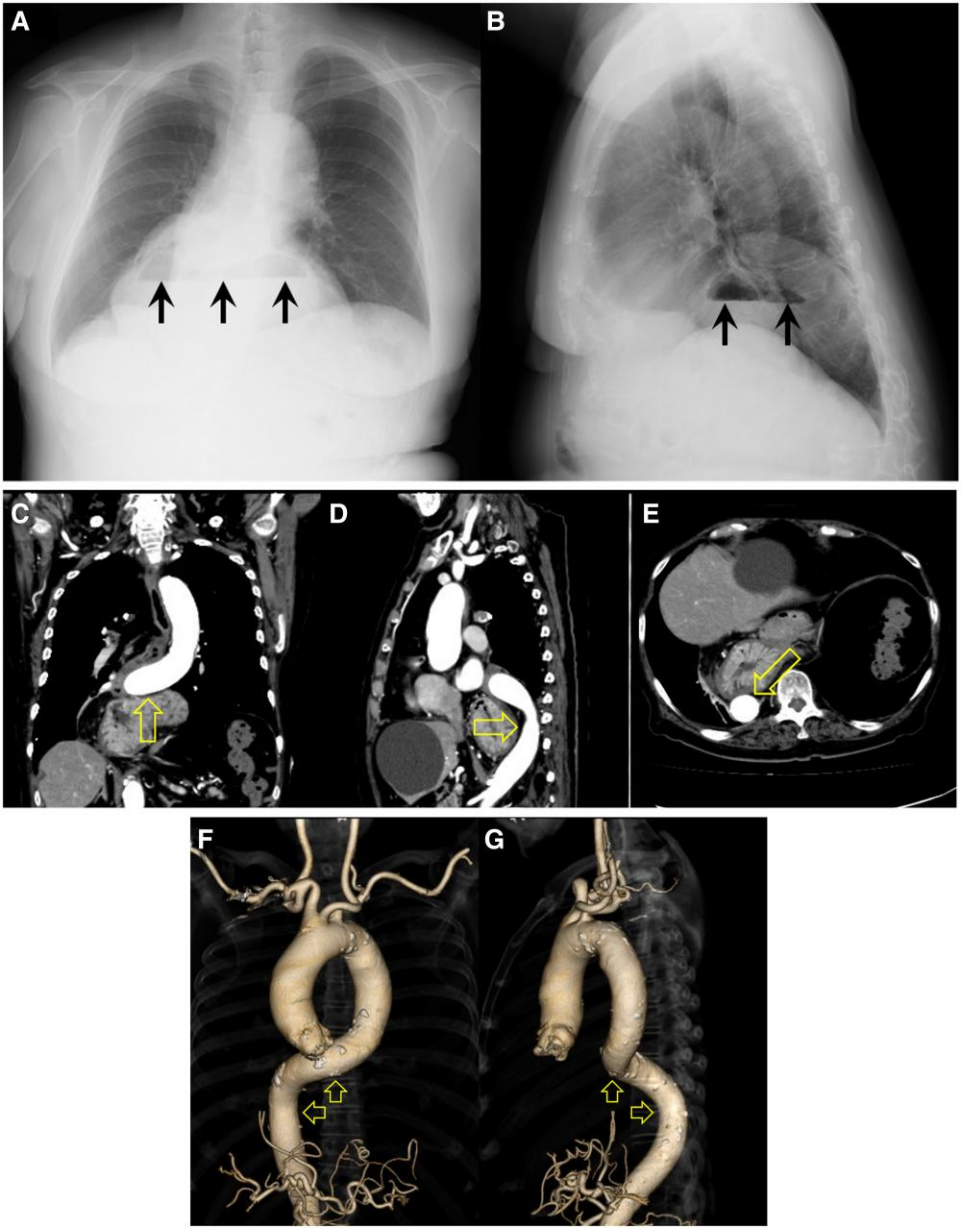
Niveaus (arrows) in the lower mediastinum on chest radiography (*A*, posterior-to-anterior; *B*, right-to-left views). The distal descending thoracic aorta deviated (open arrows) cranially (*C*, coronal plane), dorsally (*D*, sagittal plane), and dextrally (*E*, axial plane) by oesophageal hiatal hernia on contrast-enhanced computed tomography scans. The remarkably tortuous aorta compressed (open arrows) cranially/dextrally (*F,* anterior-to-posterior view) and cranially/dorsally (*G*, left-to-right view) by the hernia on 3D computed tomography aortography.
